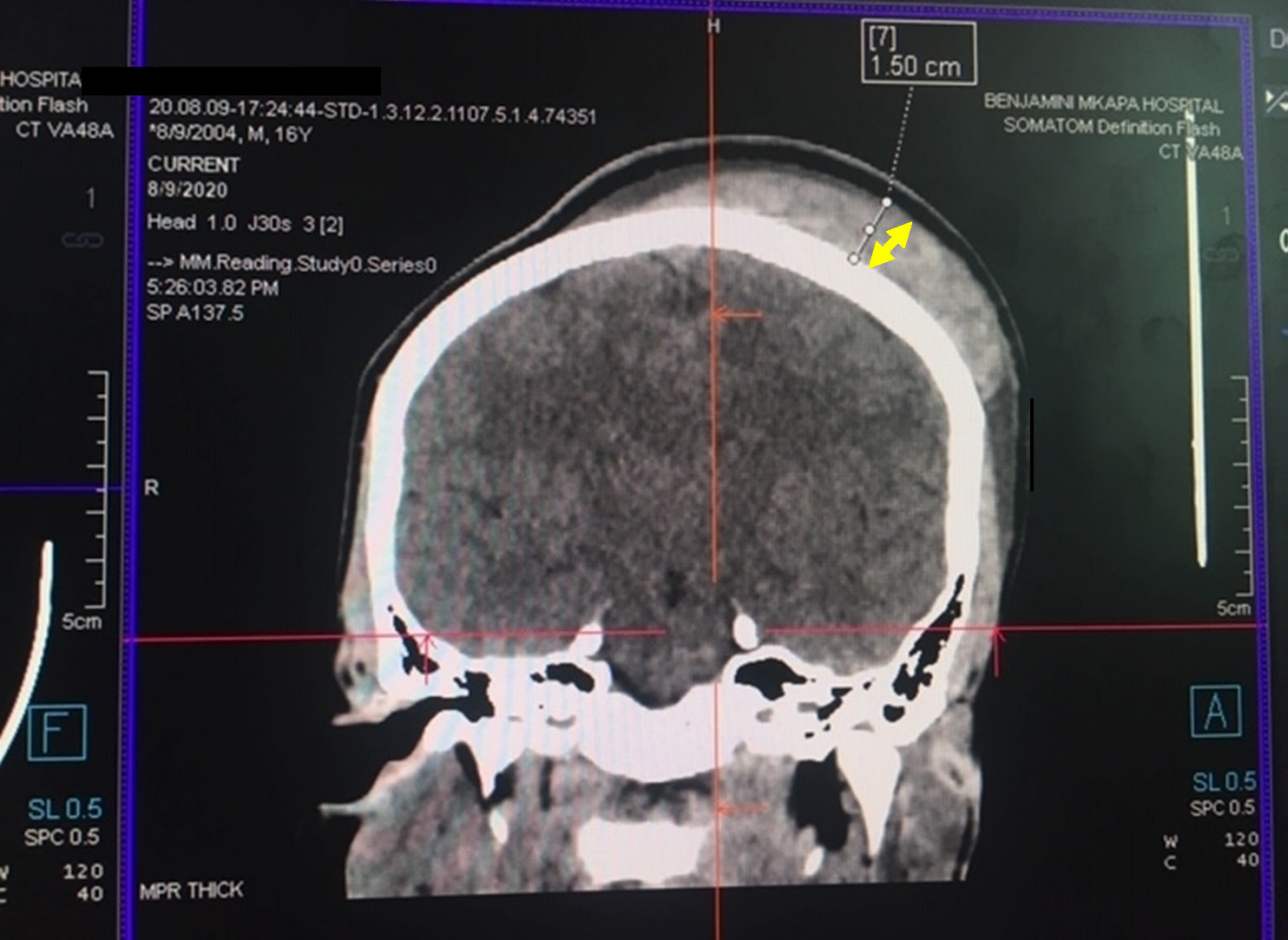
Enhanced head coronal view CT scan showing subgaleal hematoma measuring 1.5 cm (yellow arrow) overlying parietal bone with intact calvarium and normal brain parenchyma.
